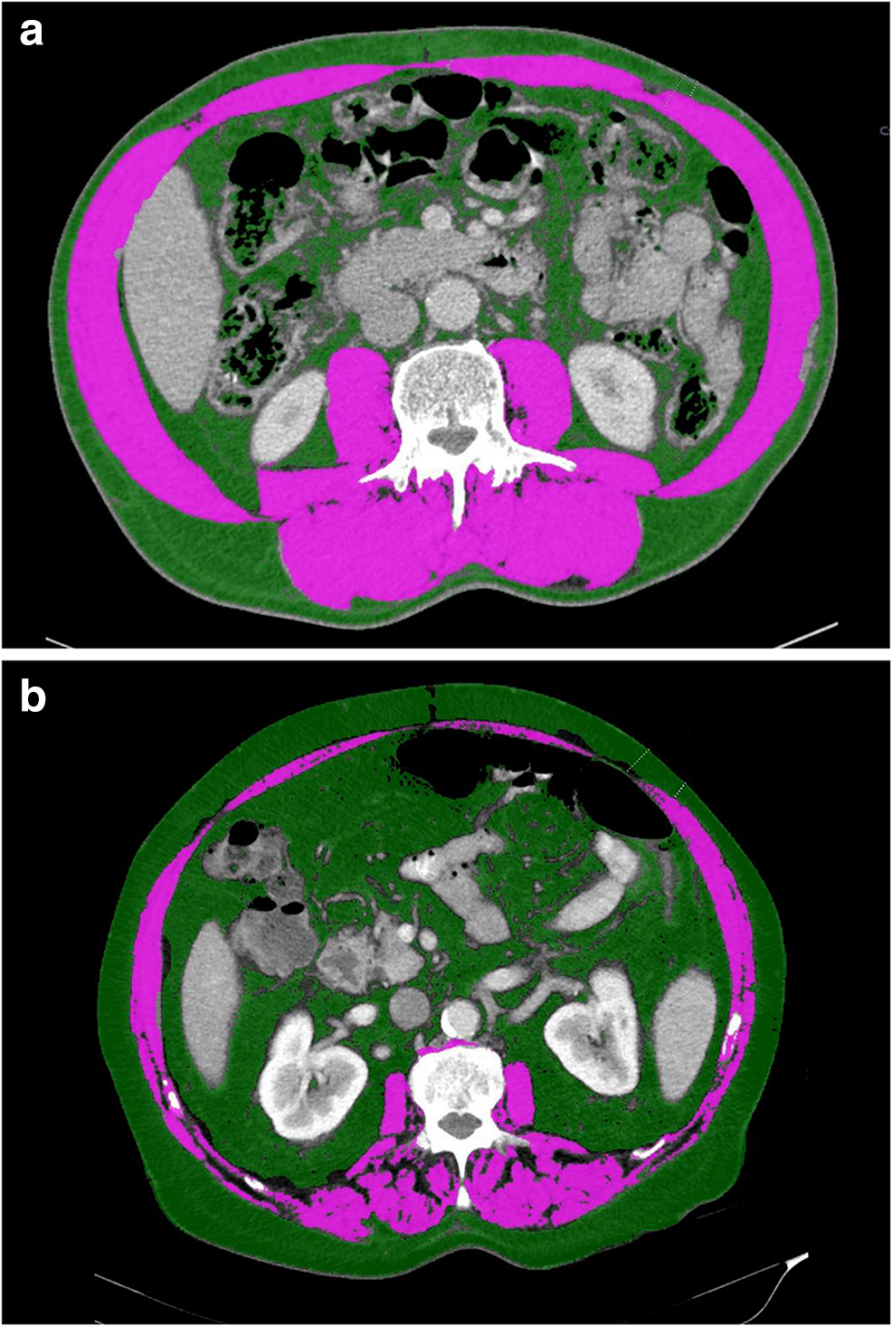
Abdominal CT images of patients with identical BMI (28.7 kg/m^2^), patient A with a SMI of 67.8 cm^2^/m^2^, and patient B with a SMI 35.3 cm^2^/m^2^; green: subcutaneous and visceral fat tissue, pink: skeletal muscle tissue
